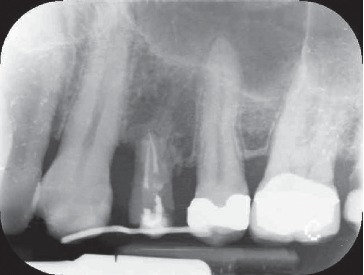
Clinical case #8: tooth 2.4, X-ray after 12 weeks of stabilization. Time: T1.
